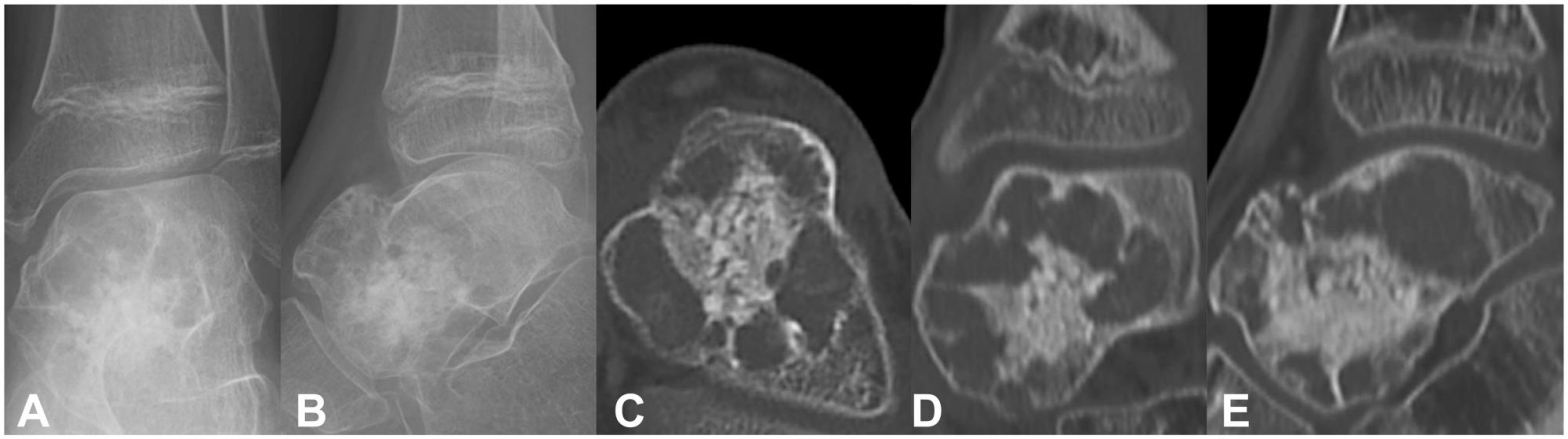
(A and B) Radiograph 4 months postoperatively revealed resorption of the artificial bone and bone translucency. (C, D, and E) CT examination 5 months postoperatively revealed a mixture of bone sclerosis and bone lucency.
